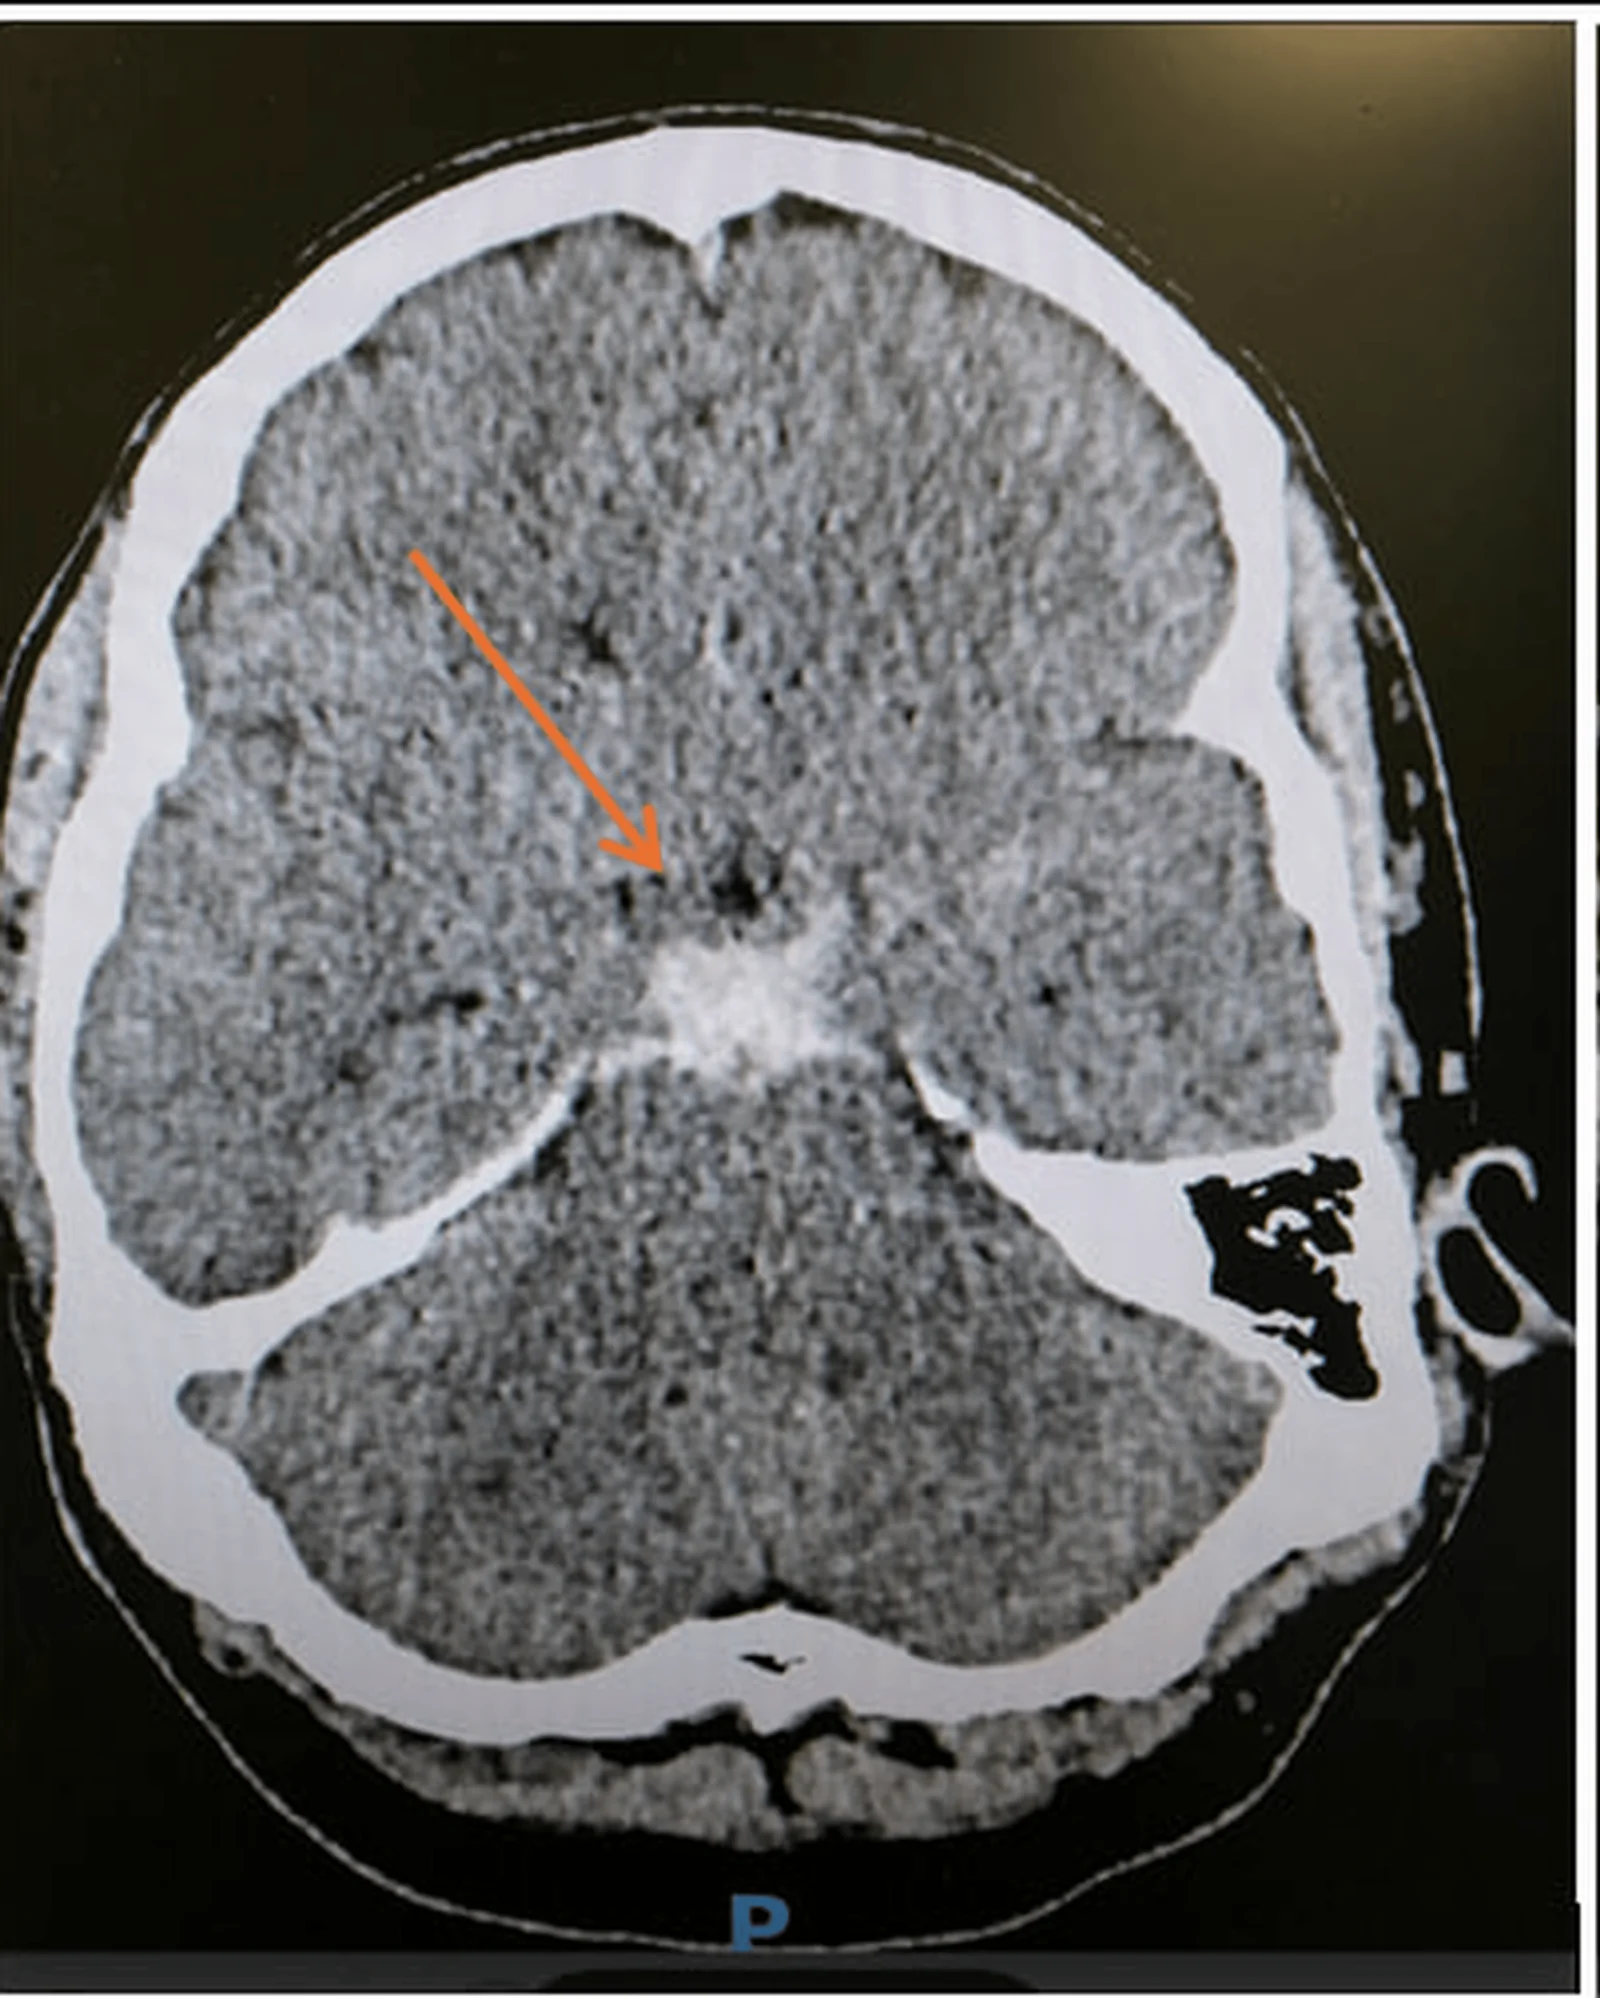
Non-contrast CT of the head showing perimesencephalic subarachnoid hemorrhage (PMSAH, arrow) confined to the prepontine and interpeduncular cisterns, consistent with the diagnosis of PMSAH.
